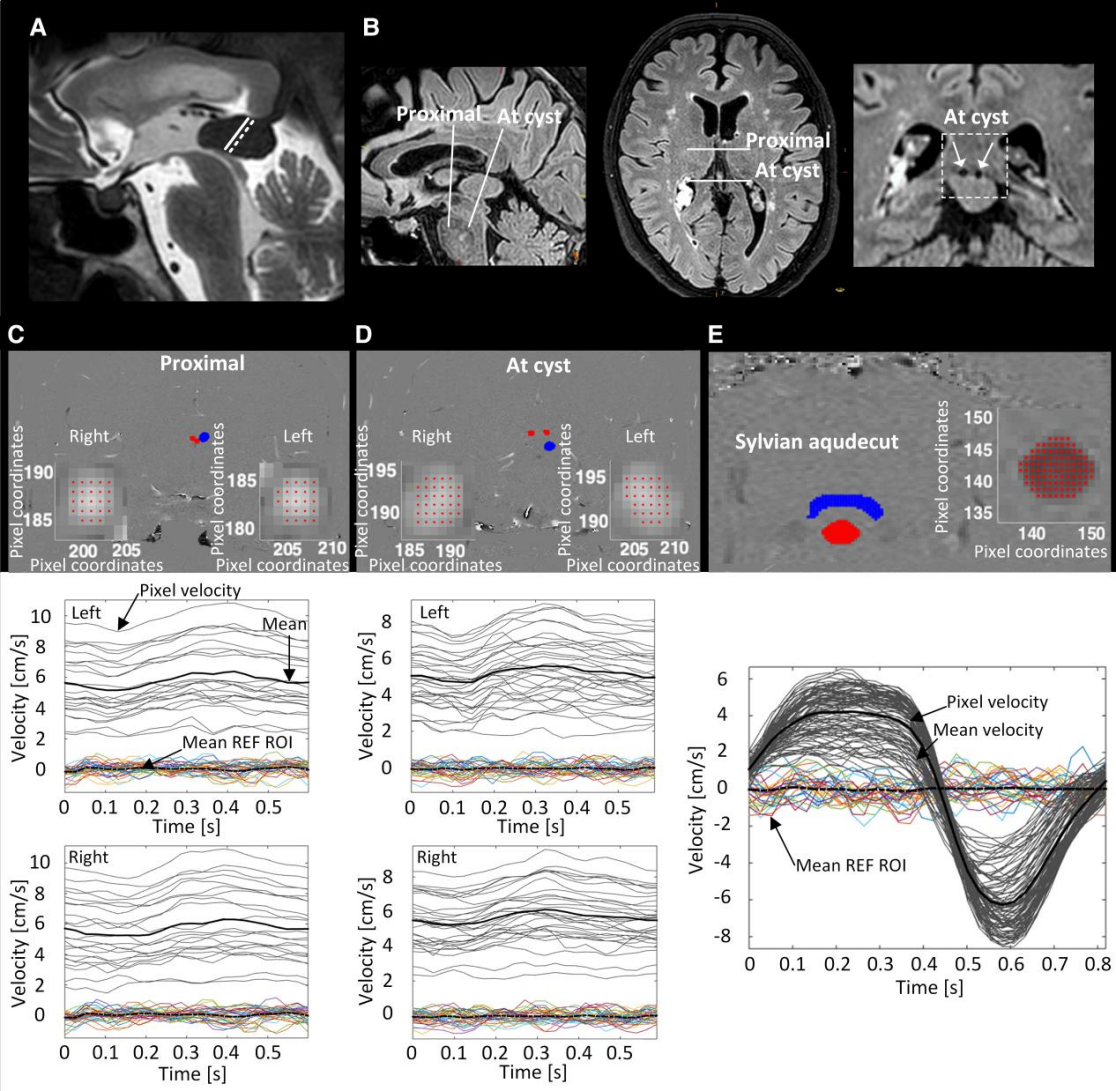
Image-based biomarkers of physiological alterations associated with crowding of the pineal recess in symptomatic patients with non-hydrocephalic pineal cysts. (A) The tectum-splenium-cyst ratio was defined from midsagittal MRI as the ratio between the pineal cyst diameter drawn at the shortest distance from the splenium of corpus callosum to the tectum divided by the length of the splenial-tectal distance drawn along the same line. (B) Blood flow in the internal cerebral veins was estimated proximal (upstream) to the cyst (proximal) and at the cyst (at cyst). (C) From phase-contrast MRI sequences, regions of interest (ROIs) were placed in the left and right internal cerebral veins (shown in red) proximal to the cyst (proximal). Reference ROIs (blue) were placed nearby. Zoom of the left vein at lower left and zoom of the right vein at lower right. Flow velocities were determined for every pixel within the ROI. Grey lines show the vein pixel velocities with black line referring to mean velocity of the vein. The coloured pixel velocities are from the reference ROI with black stippled line showing the mean velocity of the reference ROI. (D) Likewise, ROIs (red) were placed in the internal cerebral veins at the cyst (at cyst). Reference ROIs (blue) were placed nearby. Zoom of the left vein at lower left and zoom of the right vein at lower right. Flow velocities were determined for every pixel within the ROI. Grey lines show the vein pixel velocities with black line referring to mean velocity of the vein. The coloured pixel velocities are from the reference ROI with black stippled line showing the mean velocity of the reference ROI. (E) For estimation of net CSF flow in the Sylvian aqueduct, the ROI was placed within the Sylvian aqueduct (red) and the reference ROI (blue). Zoom of the Sylvian aqueduct to the right. Flow velocities were determined for every pixel within the ROI. Grey lines show the vein pixel velocities with black line referring to mean velocity of the vein. The coloured pixel velocities are from the reference ROI with black stippled line showing the mean velocity of the reference ROI.
